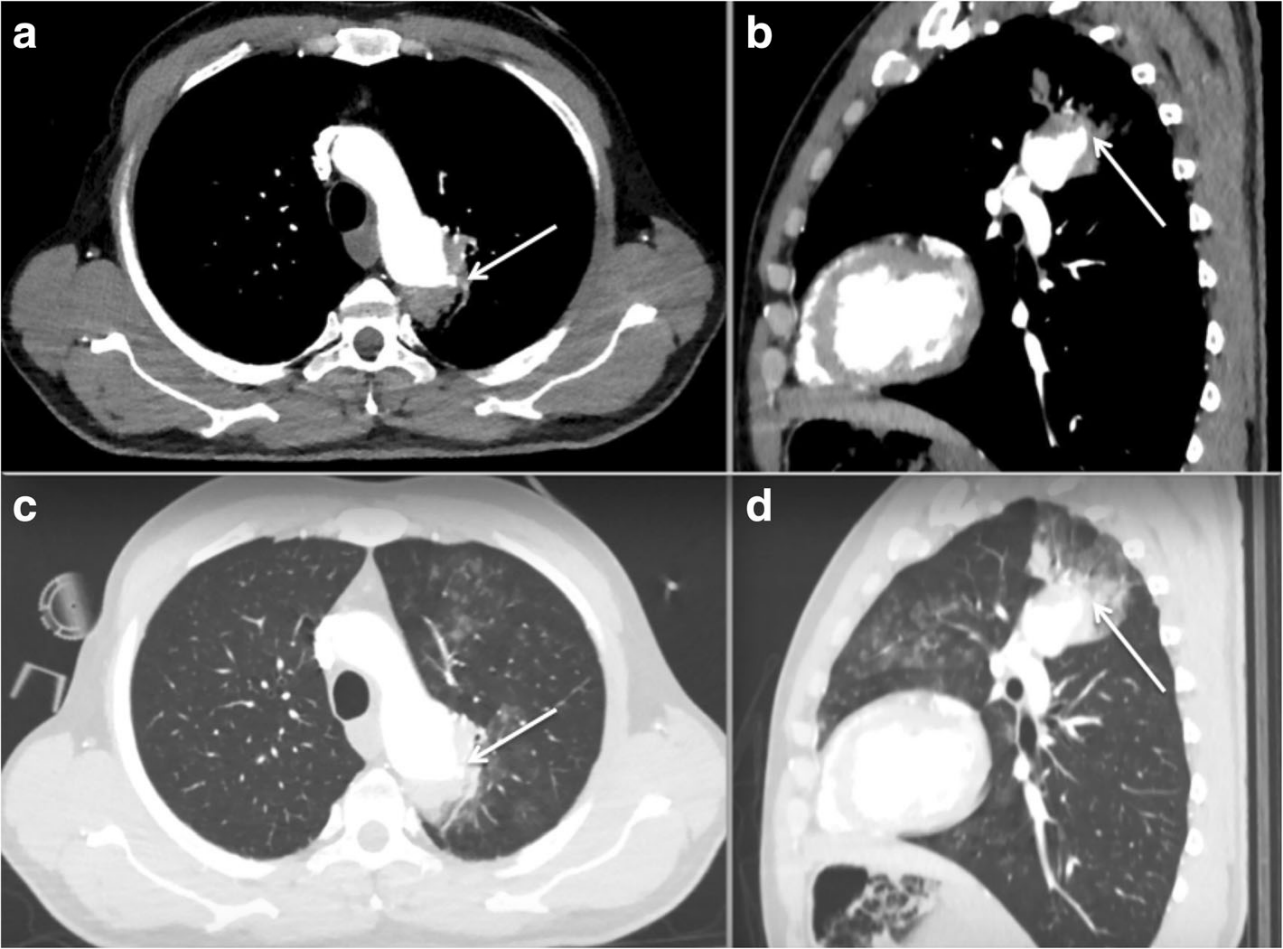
Chest CT scan images lung windows (panels a and b) and mediastinal windows (panels c and d) showing aneurysmal dilatation of thoracic aorta with upper posterior leak (arrows) and hemorrhagic parenchymal spreading
